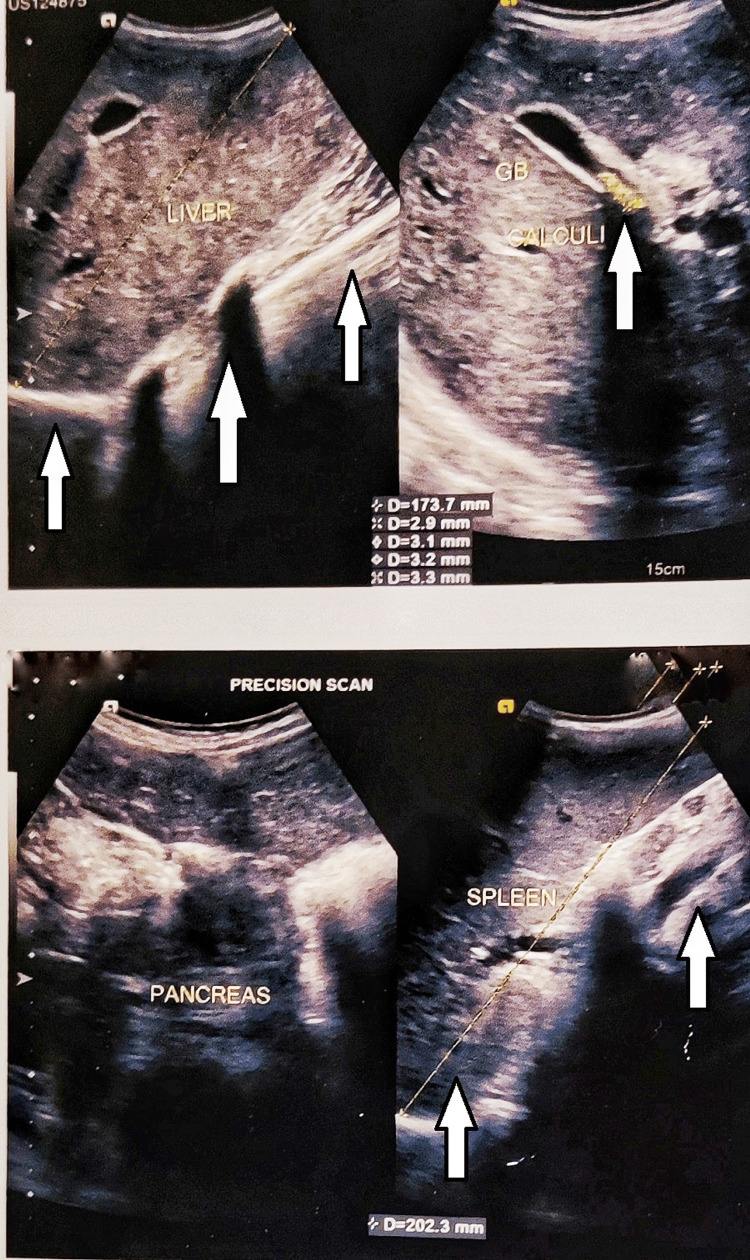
Ultrasonography of the abdomen reveals enlarged liver with a size of 17.3 cm, an enlarged spleen with a size of 20.2 cm, and multiple calculi at the neck of the gall bladder measuring 6 × 6 mm in size
